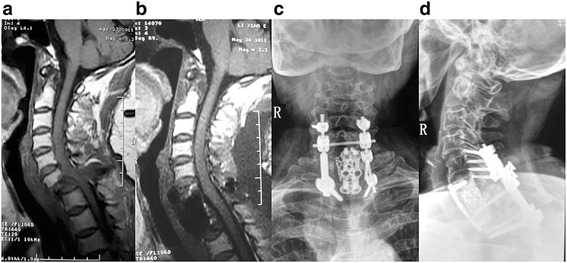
A 63-year-old female with breast cancer was admitted because of nonambulatory and severe pain. Mid-sagittal T1 MR image (a) showing C7 pathological fracture-dislocation and encroachment of spinal canal. Postoperative T1 MR image (b) obtained after combined posterior resection, anterior corpectomy, and reconstructive surgery, showing the spinal canal was significantly decompressed. Postoperative anteroposterior (c) and lateral (d) radiographs showing the good spinal alignment achieved after surgery. The patient regained ambulatory and self-care ability
